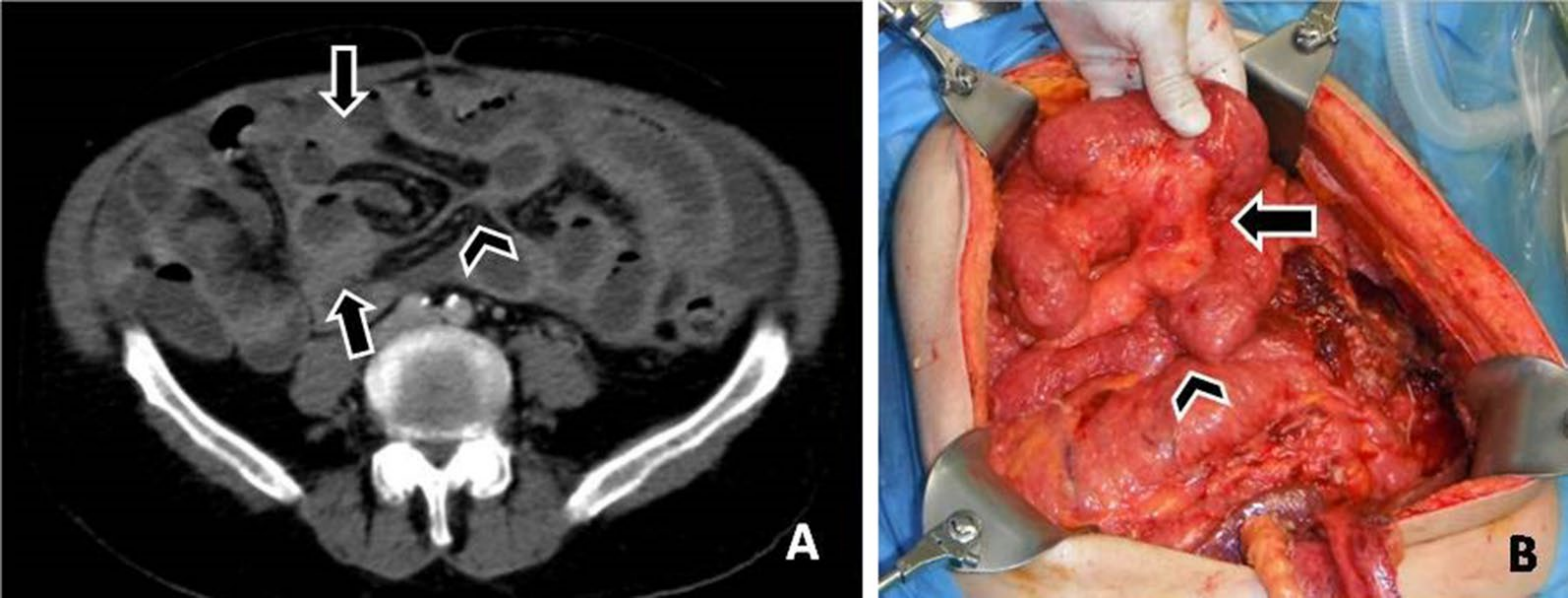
a CT enteroclysis image demonstrates extensive wall thickening of the small bowel (arrows)—“layered type” of SB involvement and thickening of the mesenteric surface (arrowhead). b. Corresponding surgical imaging
